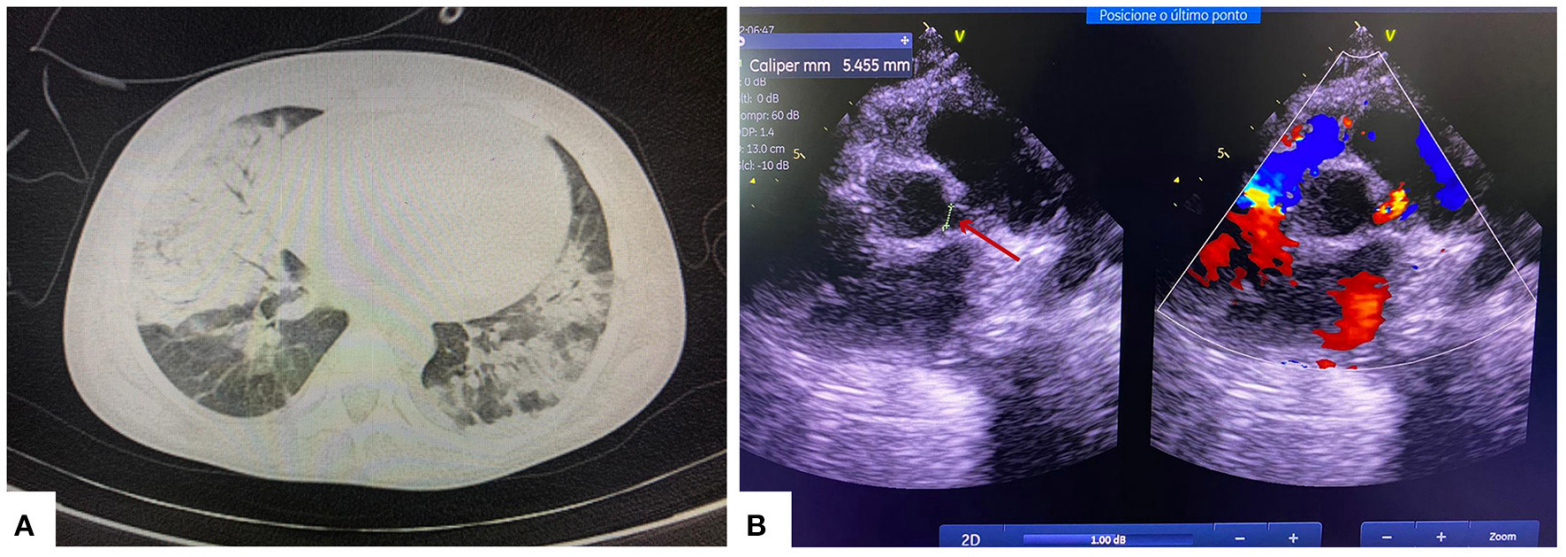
(A) Chest computed tomography showing multifocal and confluent consolidations with ground-glass attenuation in >50% of the pulmonary parenchyma. (B) Echocardiogram showing left coronary artery dilatation (red arrow).
